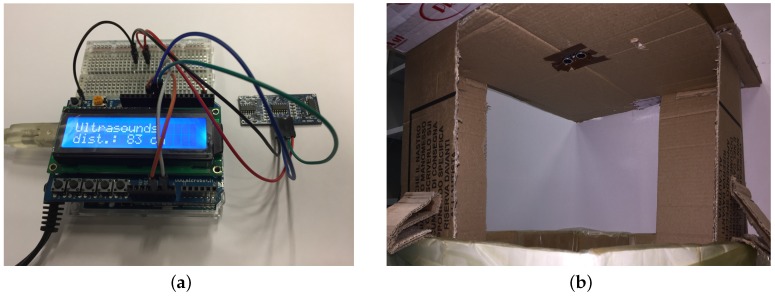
Experimental setup: (a) the LCD screen showing a measured distance value; (b) the ultrasound sensor placed in the cardboard cover.
